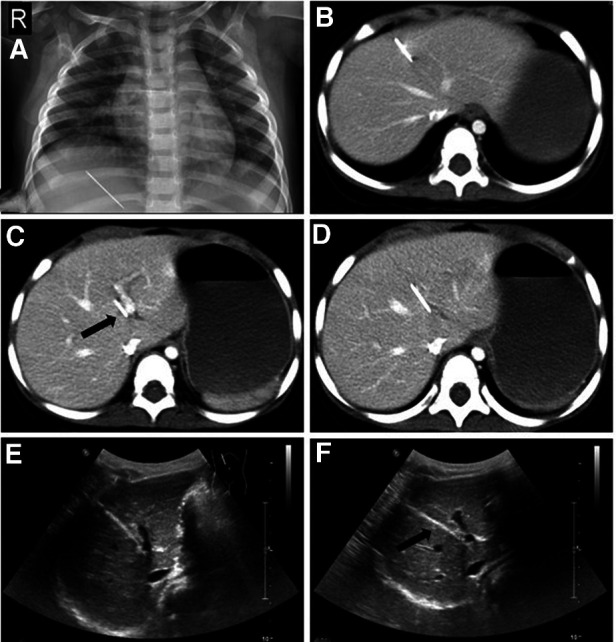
Preoperative imaging examinations of the patient. (A) An accidental chest x-ray showing a striped high-density shadow in the hepatic region. (B–D) Contrast-enhanced CT showing the major part of the sewing needle trapped in the liver parenchyma with the tip closest to the sagittal part of the left branch of the portal vein. (E,F) Ultrasound images showing a minimal distance between the sewing needle and portal vein.
